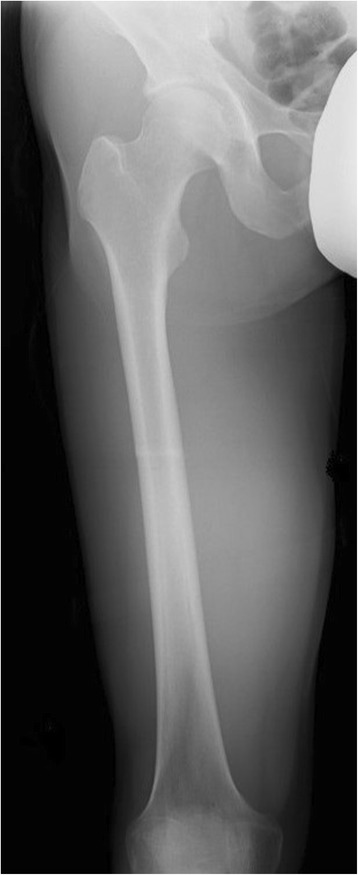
Radiography reveals a soft tissue mass with no calcification or periosteal reaction
